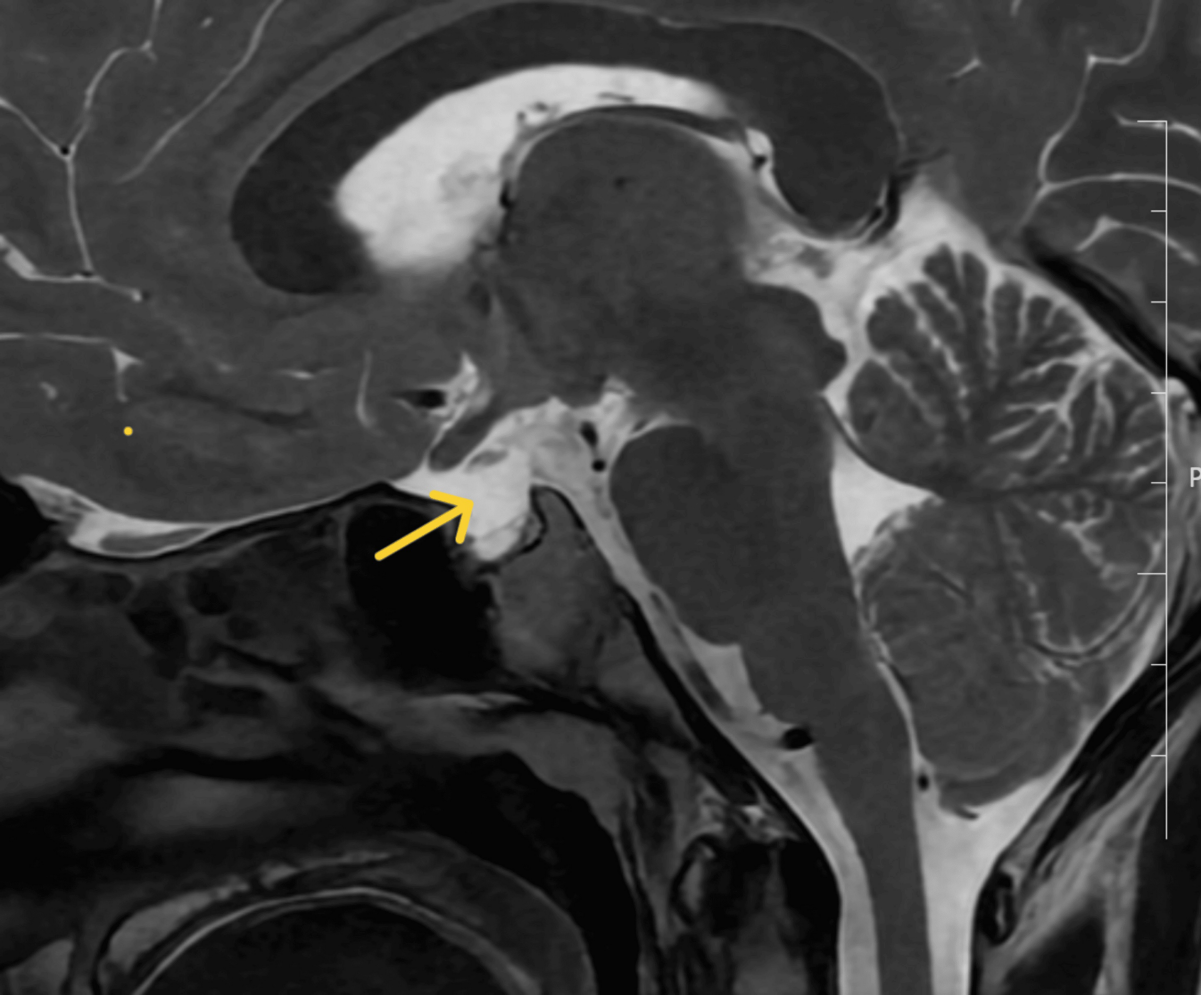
Sagittal T2-weighted MRI of sella turcica showing absent pituitary stalk (yellow arrow) of Case 2
